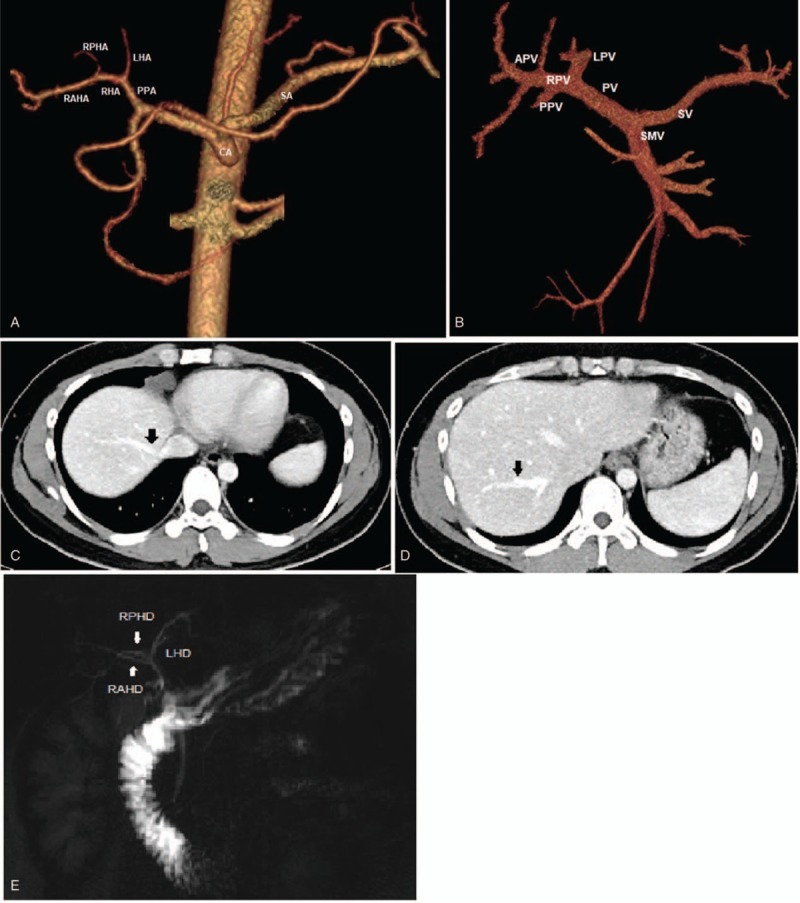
Preoperative imaging studies. Computed tomography (CT) scan three-dimensional reconstruction showing a normal variant of the hepatic artery (A) and portal vein (B). The tributaries of the right hepatic vein (RHV) (black arrows) draining segment VII (C) and segment 5 (D). (E) The trifurcation of the biliary tree was noted on MRCP.
